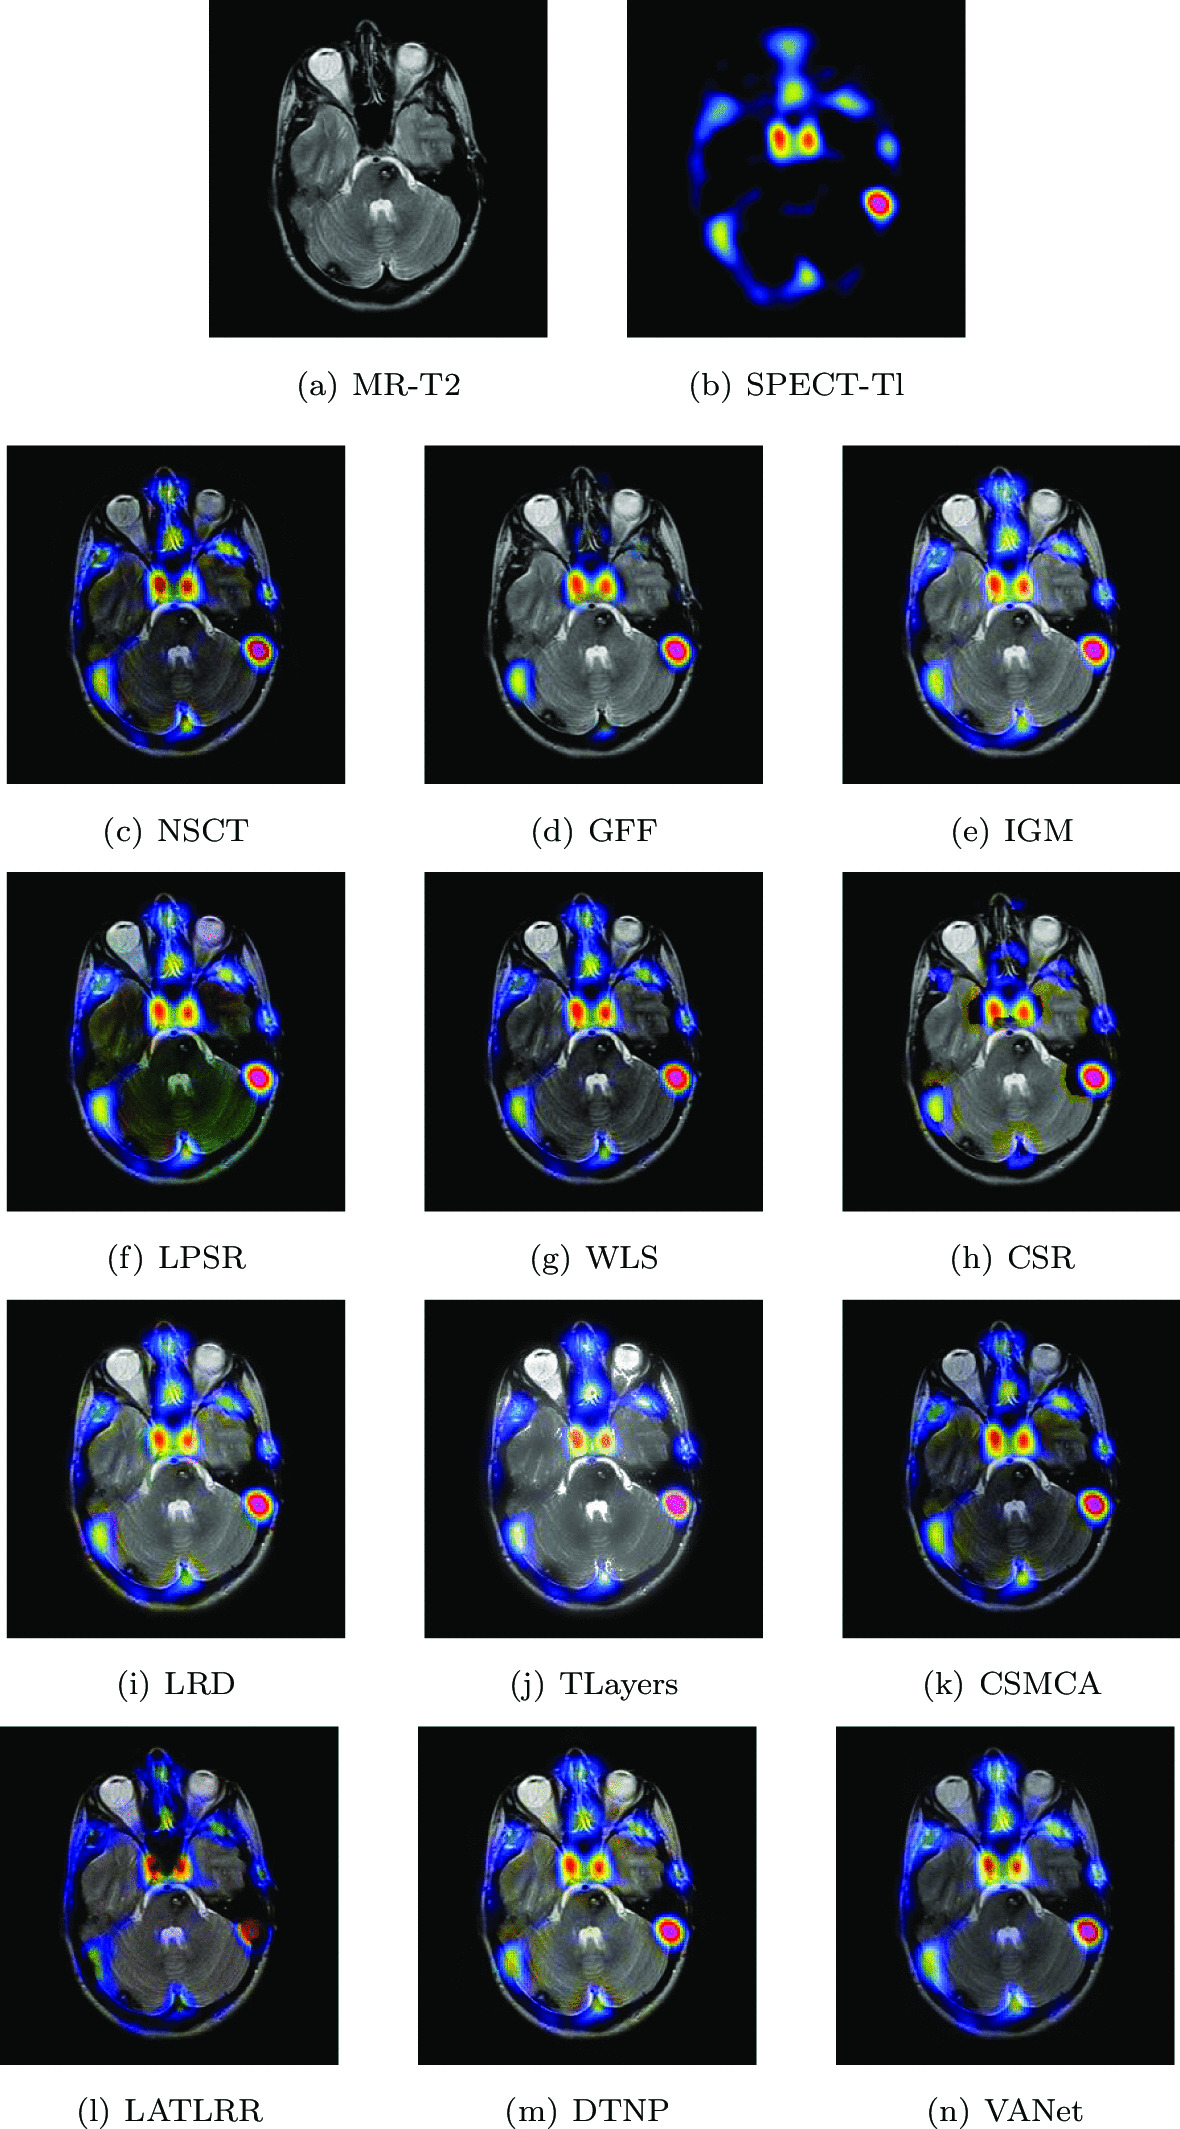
The first set of fused MRI-SPECT images from 9 methods on cavernous angioma
